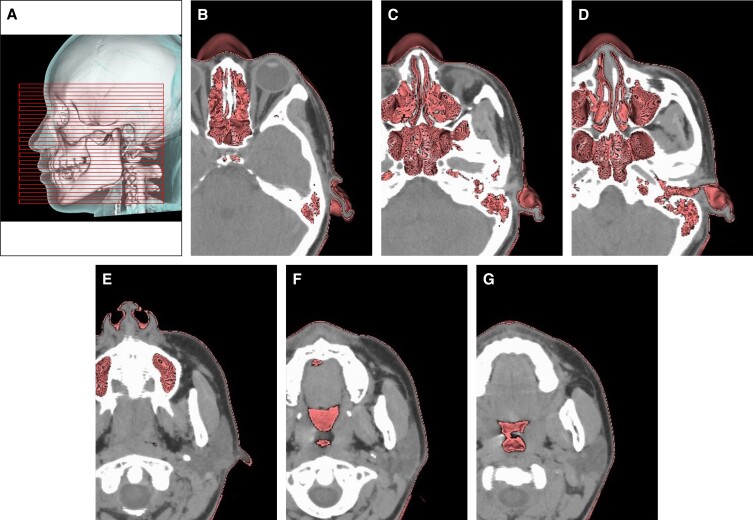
Creation of axial and coronal computed tomography (CT) images. (A) A leading three-dimensional CT(3D CT) image for accurately generating axial CT images. An infraorbitomeatal (IOM) line drawn between the infraorbital margin and external acoustic meatus is a global standard reference for CT. Axial CT images on lines parallel to the IOM line can be accurately created while simultaneously checking the existing anatomical structure. Coronal CT images were created in a plane orthogonal to axial CT images. (B-G) A guideline for creating accurate axial CT images. Axial CT images were cut from the whole-facial 3D CT image and a combination of several axial images of the left halves of the face. The accuracy of the axial CT images for each participant was confirmed using this reference image. The positional relationship between the structures of the facial surface and structures under the skin can be easily comprehended, and the superficial musculoaponeurotic system (SMAS) is visible as a linear structure in the subcutaneous adipose tissue. Its thickness varies depending on the facial region. (B) At the level of the central part of the eyeball: ethmoidal sinus is depicted. In the subcutaneous adipose tissue of the temporal region, membranous structures are visible. In order from the surface, the SMAS, superficial temporal fascia, and deep temporal fascia covering temporal muscle are distributed in layers. (C) At the level of the lower part of the eyeball: the maxillary and sphenoid sinuses are visible. In the subcutaneous adipose tissue of the temporal region, a membranous structure, the SMAS is recognized. (D) An image on IOM: the lower edge of the orbit, auricle and external acoustic meatus are visible. In the subcutaneous adipose tissue of the upper cheek, the SMAS is recognized. (E) At the level of the lower edge of the nose: the zygomatic major muscle and the anterior margin of the parotid gland are visible, and the SMAS is recognized in the subcutaneous adipose tissue between them. The masseter muscle exists deeply them. (F) At the level of the upper mandibula: the SMAS is recognized between the zygomatic major muscle and parotid gland. (G) At the level of the corner of the mouth: the SMAS is recognized between the major zygomatic muscle attaching to the orbicularis oris muscle and parotid gland.
